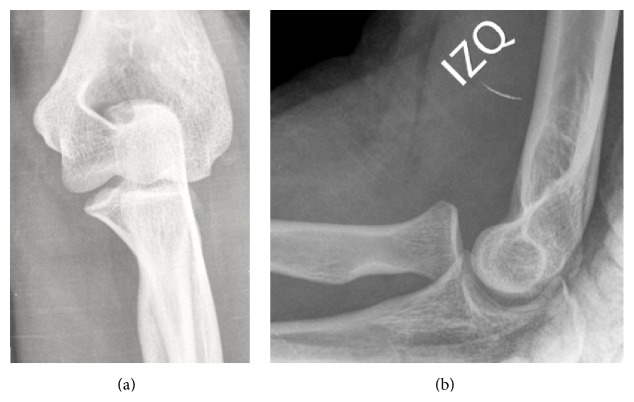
Left elbow anteroposterior (a) and lateral (b) X-ray where the medial and anterior dislocation of the radial head can be observed. No pathology is observed on the ulnohumeral joint.
